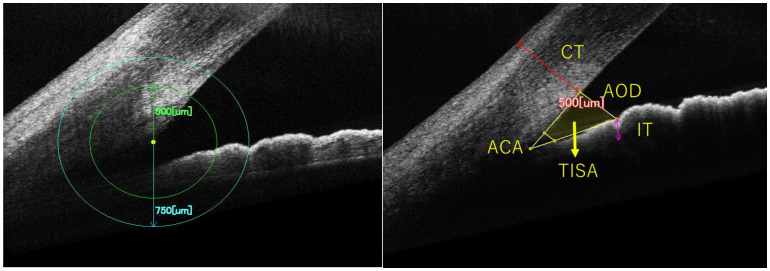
Definition of Anterior Chamber Angle Configuration Parameters Using OCT: A Right Eye Example. ACA, AOD, TISA, PIT, PCT were measured at 500 μm and 750 μm from the scleral spur. ACA: the anterior chamber angle, AOD: angle opening distance, TISA: trabecular iris space area, IT: iris thickness, CT: corneal thickness.
